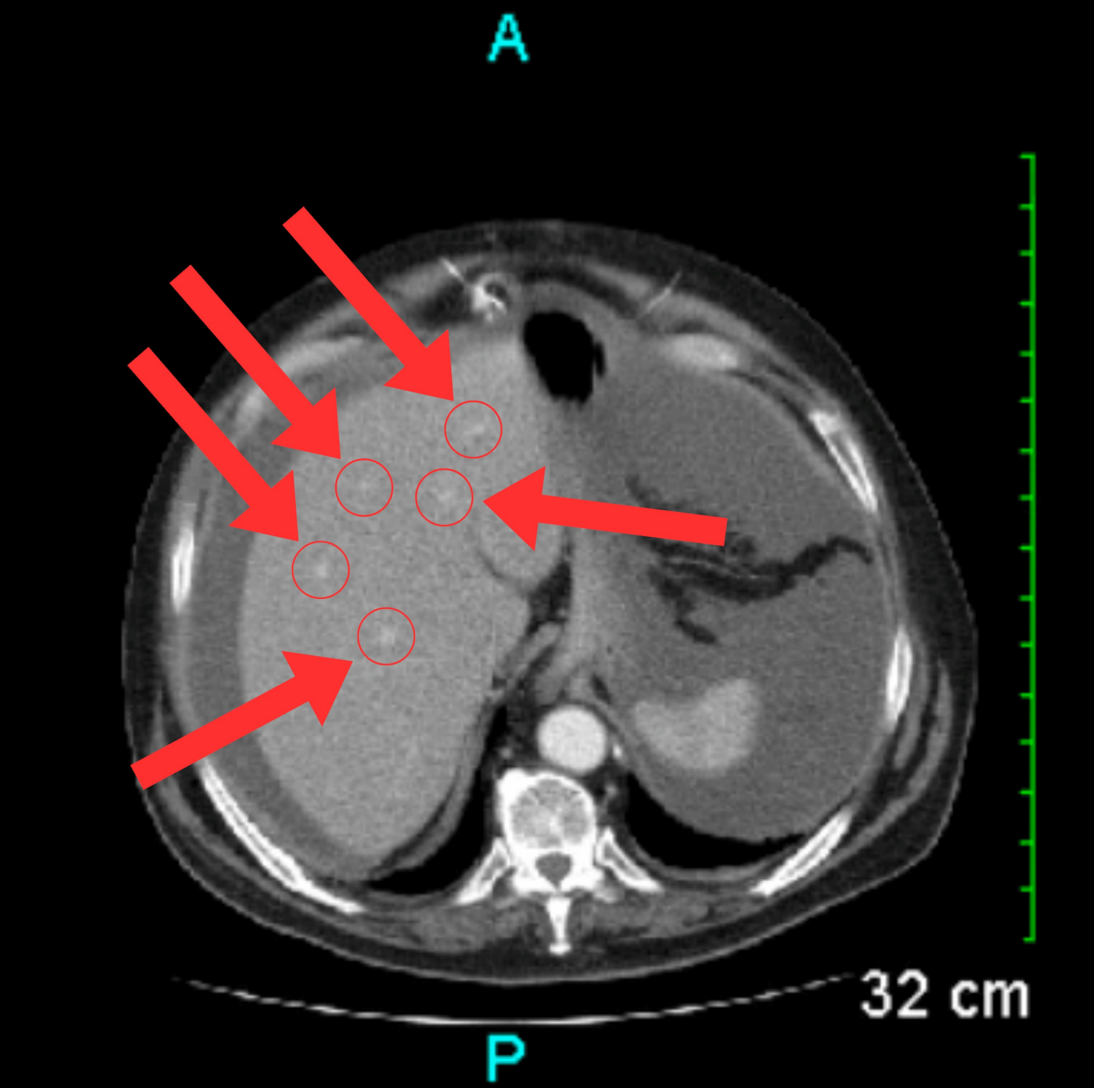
CT scan showing mild hepatic steatosis (red arrows and circles). CT: Computed tomography
